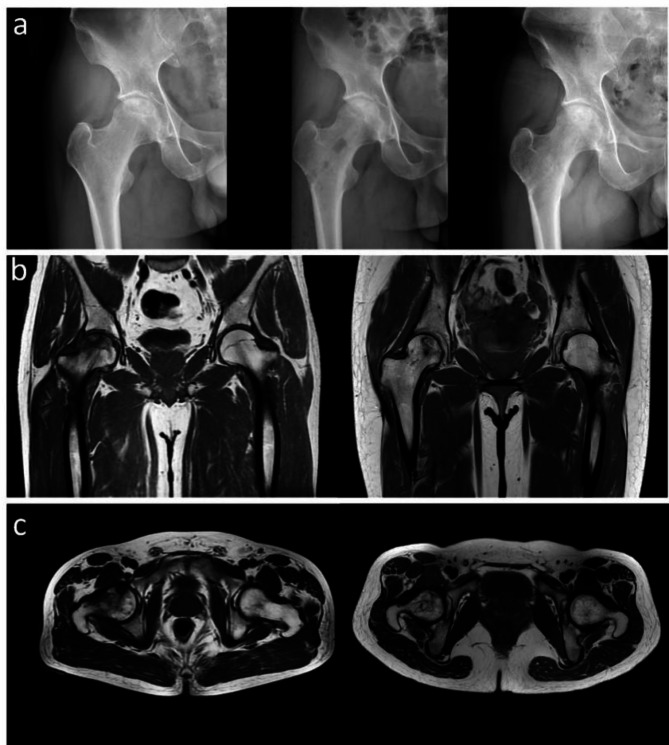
(a) X-rays of pre-operation, 3months after operation, and 9 months after operation; (b) Coronal MRI of pre-operation and 9 months after operation; (c) Cross-sectional MRI pre-operation and 9 months after operation.
